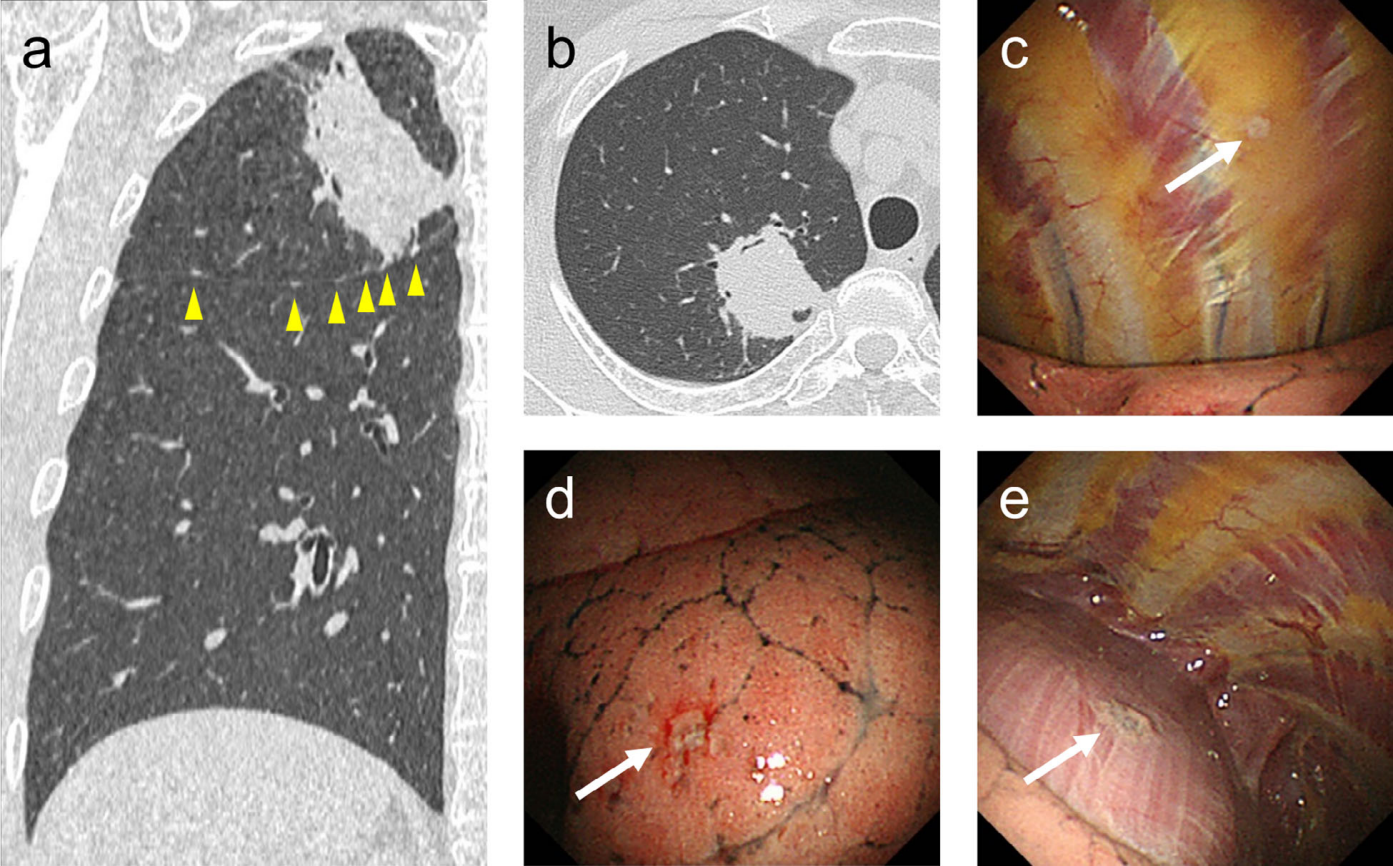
A case of dry pleural dissemination in a 69‐year‐old woman (case 4 in Table 2); (a) coronal and (b) axial sections of a computed tomographic scan (lung window) show a primary lesion abutting the right major fissure in the right upper lobe, pleural nodules only on the fissure (arrow head), and no pleural effusion. Local anesthetic thoracoscopic image reveals a small number of tiny, flat, and glossy nodules (arrow) without pleural effusion on the (c) parietal, (d) visceral, and (e) diaphragmatic pleura
